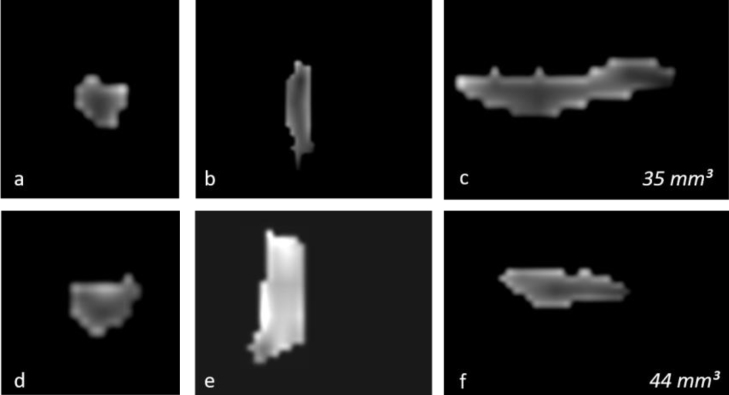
Changes in the three-dimensional volume measurement of the left OB in a total laryngectomized patient on magnetic resonance images, before olfactory rehabilitation (a–c, upper row) and after olfactory rehabilitation (d–f, lower row), as coronal (a, d), axial (b, e), and sagittal (c, f) images, respectively. The left OB volume was 35 mm^3^ and 44 mm^3^ before and after olfactory rehabilitation.
